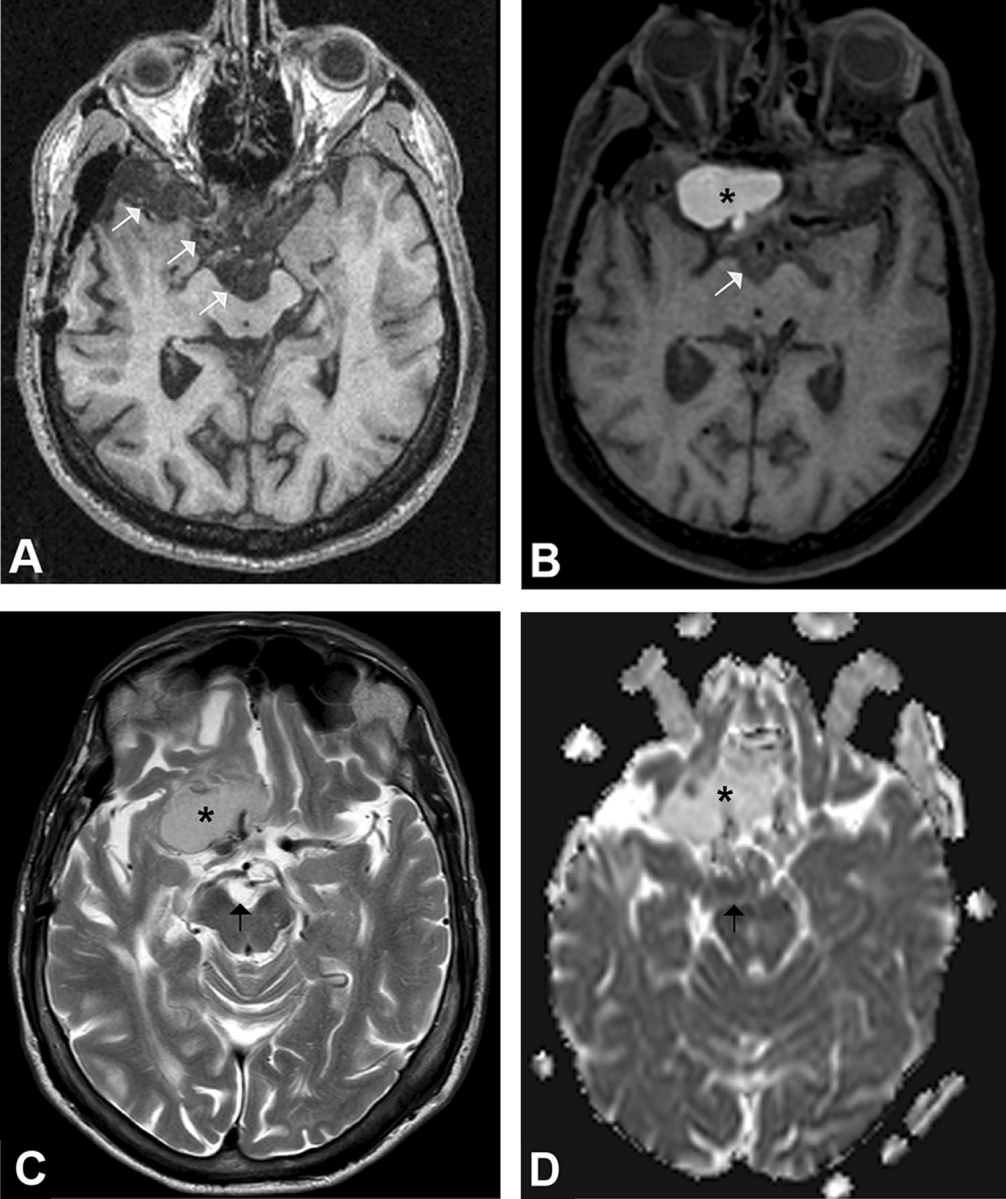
(A,B) Axial nonenhanced T1-weighted MRI in patient 3, which shows evidence of transformation of a residual classic epidermoid cyst in the suprasellar cistern and right middle cranial fossa (white arrows in A) to a white epidermoid (black asterisk in B). The interpeduncular component retained its classis epidermoid features (white arrow in B). The 2 images are taken 10 years apart. (C,D) Axial T2WI and ADC map demonstrating different signal intensities of the white epidermoid component (black asterisk) and classic epidermoid cyst (black arrow). The white epidermoid cyst shows intermediate signal intensity on T2WI and high signal intensity on the ADC map, indicating lack of diffusion restriction, in contrast to the classic epidermoid, which demonstrates high T2 signal intensity and diffusion restriction.
